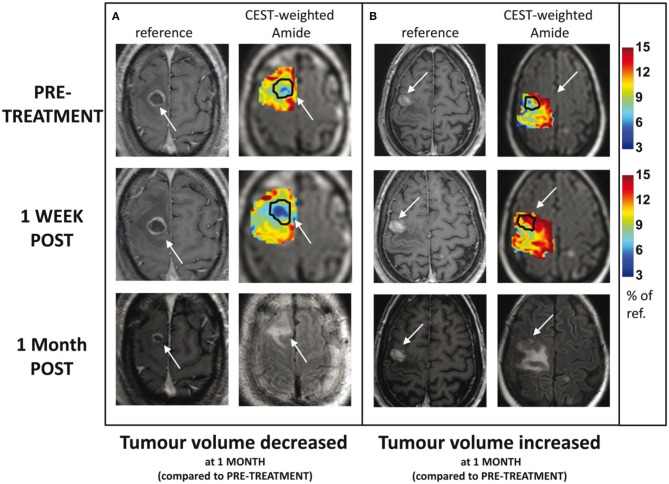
Example CEST amide effect maps within the tumor and immediately surrounding tissue, including edema, at baseline and 1 week post-treatment for 2 patients: (A) tumor volume decreased 1 month post-therapy and (B) tumor volume increased 1 month post-therapy. The margins of the enhancing tumor are indicated with an arrow and outlined in black on the CEST maps. The corresponding slice from the high resolution, contrast-enhanced T1-weighted volume is shown for comparison. Contrast-enhanced T1-weighted and FLAIR images at 1 month follow-up are shown in the third row. Reproduced, with permission, from Desmond et al. (48).
